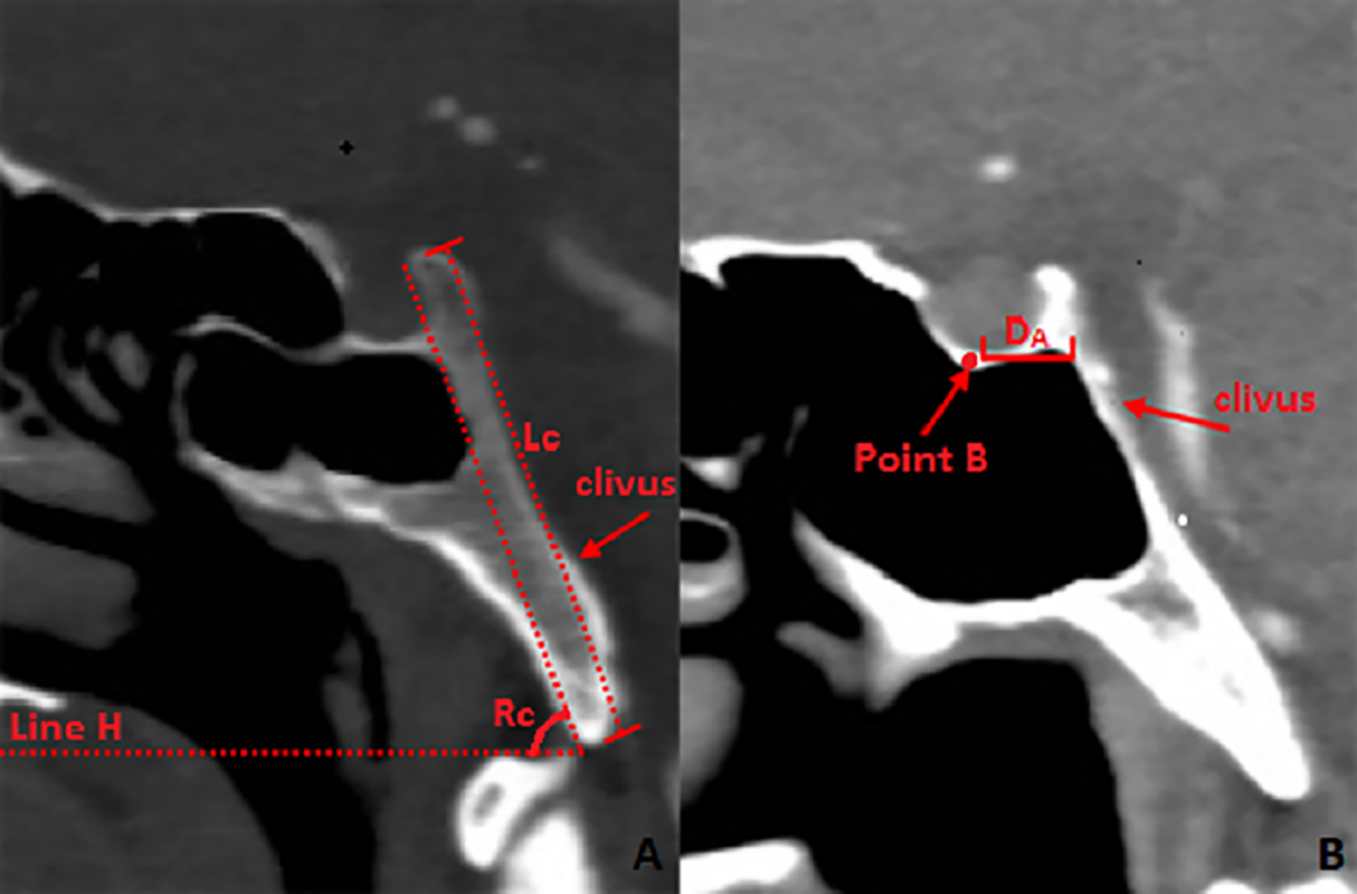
Measurement of the clivus in the sagittal plane of the CT image. 1A: Measurement of the length and angle clivus in sagittal plane in CT image (bone window); Rc: The angle between the clivus and the horizontal plane; Lc: the length of the clivus; Line H: The horizontal line. 1B: Distance from the lowest point of the sellar floor (SF) to the clivus and vertebral artery in CT image (soft-tissue window): D
